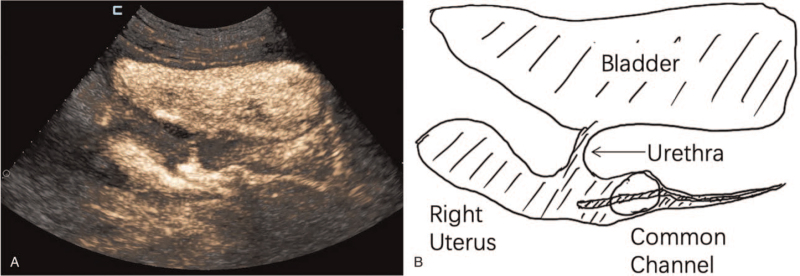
Contrast-enhanced 2-dimensional transabdominal ultrasonography showing the enhancement of the urethra, bladder, and vagina.
